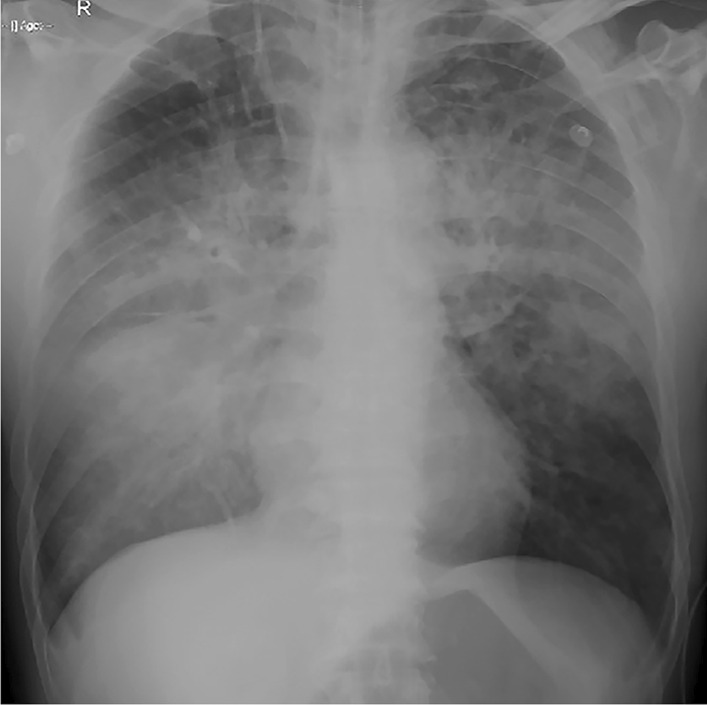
Emergency bedside chest radiography showing features of pulmonary edema with a normal cardiac silhouette during the ICU period
